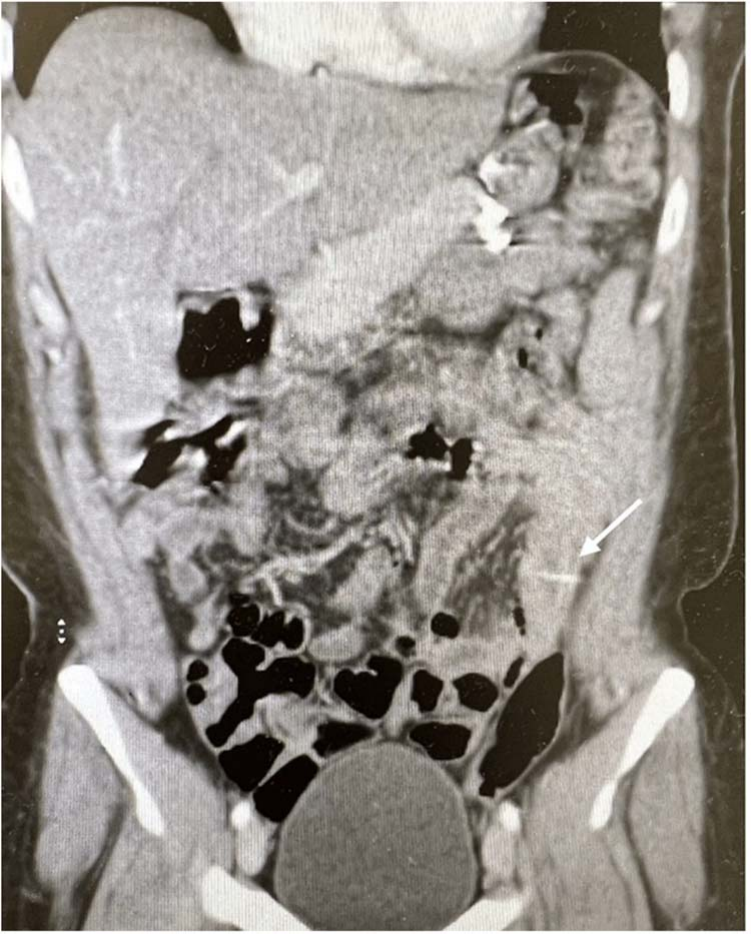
Coronal views of contrast-enhanced CT abdomen showed: a transversely oriented linear hyperdense FB (arrow) structure seen within the ilea loops in the lower quadrant.
